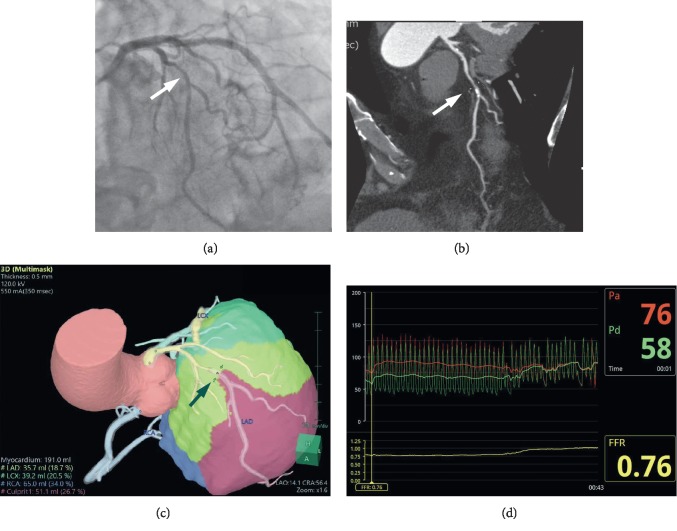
(a) Left coronary angiography (left cranial view) and (b) computed tomography angiography showed a calcified lesion (arrows) at the midsegment of the left anterior descending artery; the minimal lumen diameter (MLD) was 1.39 mm. (c) After setting a specific point at the culprit lesion (arrow), the software program (Synapse Vincent, Fujifilm Corp., Japan) showed that the myocardial mass at risk (MMAR) was 51.1 ml and that the %MMAR was 26.7%. Thus, the MMAR/MLD value was 36.8, which was highly predictive of fractional flow reserve (FFR) ≤0.8. (d) The invasive measurement revealed that the FFR was 0.76.
